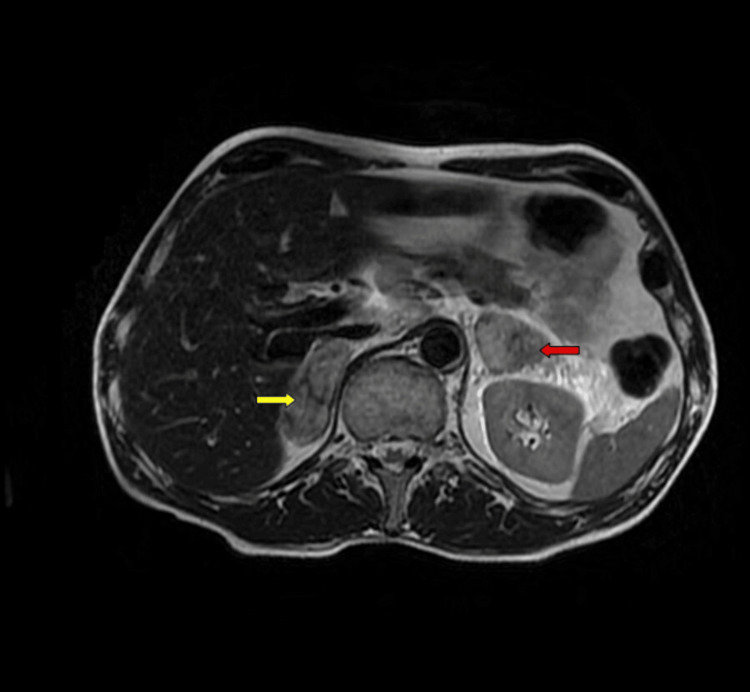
T2 MR imaging with contrast: mixed enhancement and non-enhanced center, particularly in the left adrenal gland (yellow and red arrows)
